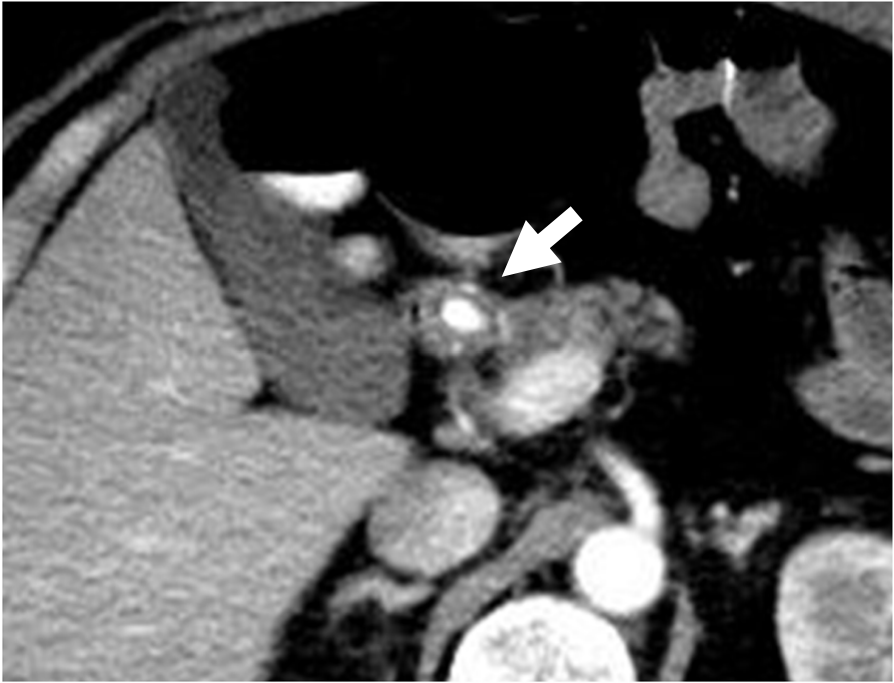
Abdominal dynamic contrast-enhanced computed tomography (CT) findings. Dynamic CT showing a pseudoaneurysm arising from the proximal gastroduodenal artery (arrow)
